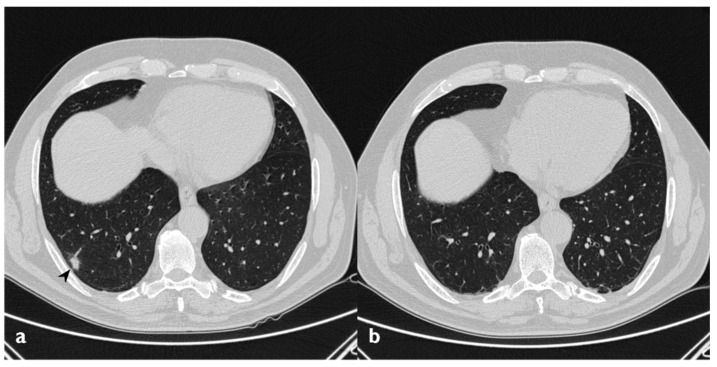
On the left (a): baseline HRCT; peripheral solid nodule in the right lower lobe (arrowheads). On the right (b): HRCT after oral corticosteroid (OCS) therapy; the previous nodule is no longer present.
